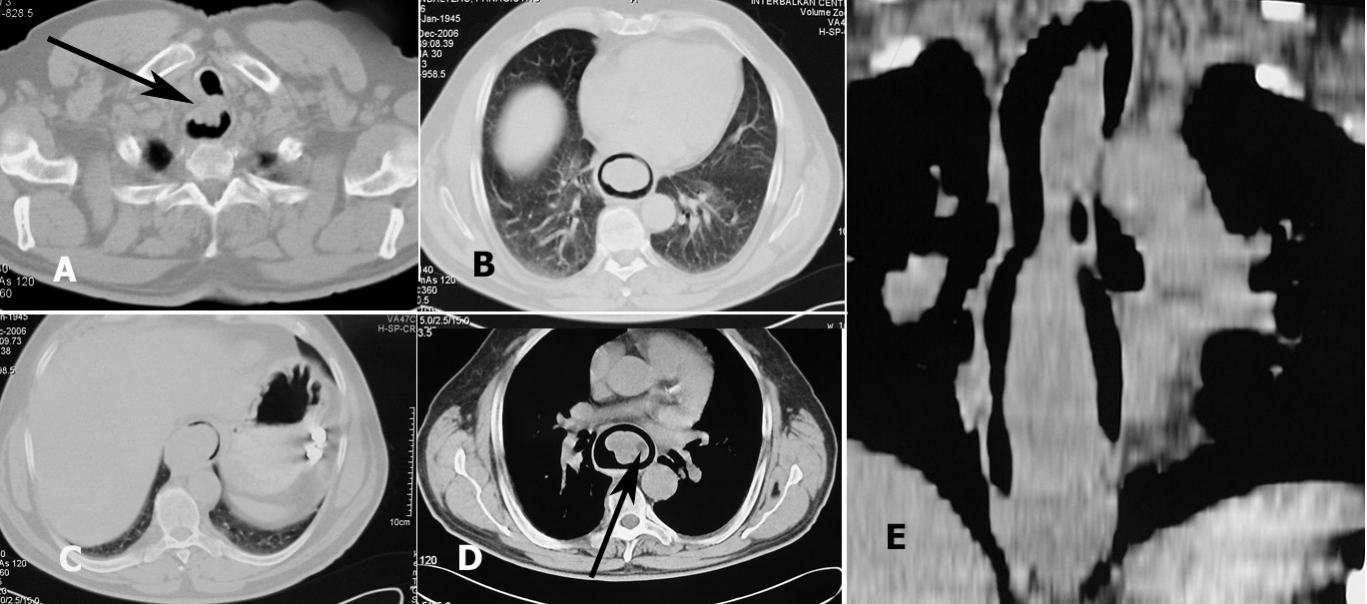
Serial axial images of the chest computed tomography scan (a,b,c,d) from the level of upper oesophagus to the level of the stomach reveal a soft-tissue, large intraluminal lesion. The polyp originates at the anterior wall of the cervical oesophagus (arrow in a). Axial computed tomography image (mediastinal window setting) shows an area of fat density (arrow in d). Reformatted coronal computed tomography image shows the entire length of the polyp (e).
